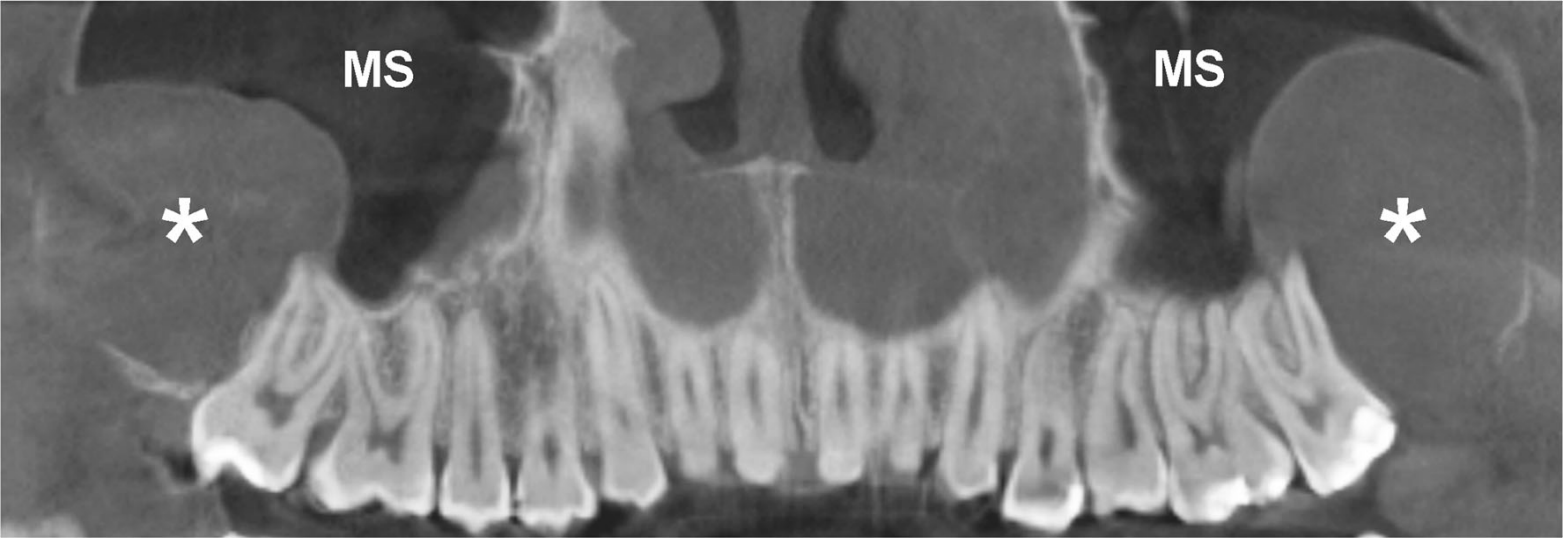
Panoramic CBCT image (1 mm thick) shows two unilocular OKCs on both sides of the maxilla (*asterisks*) in a young male patient with nevoid basal cell carcinoma syndrome (NBCCS). Both lesions, located in the posterior sextants, extend into the maxillary sinuses (*MS*)
